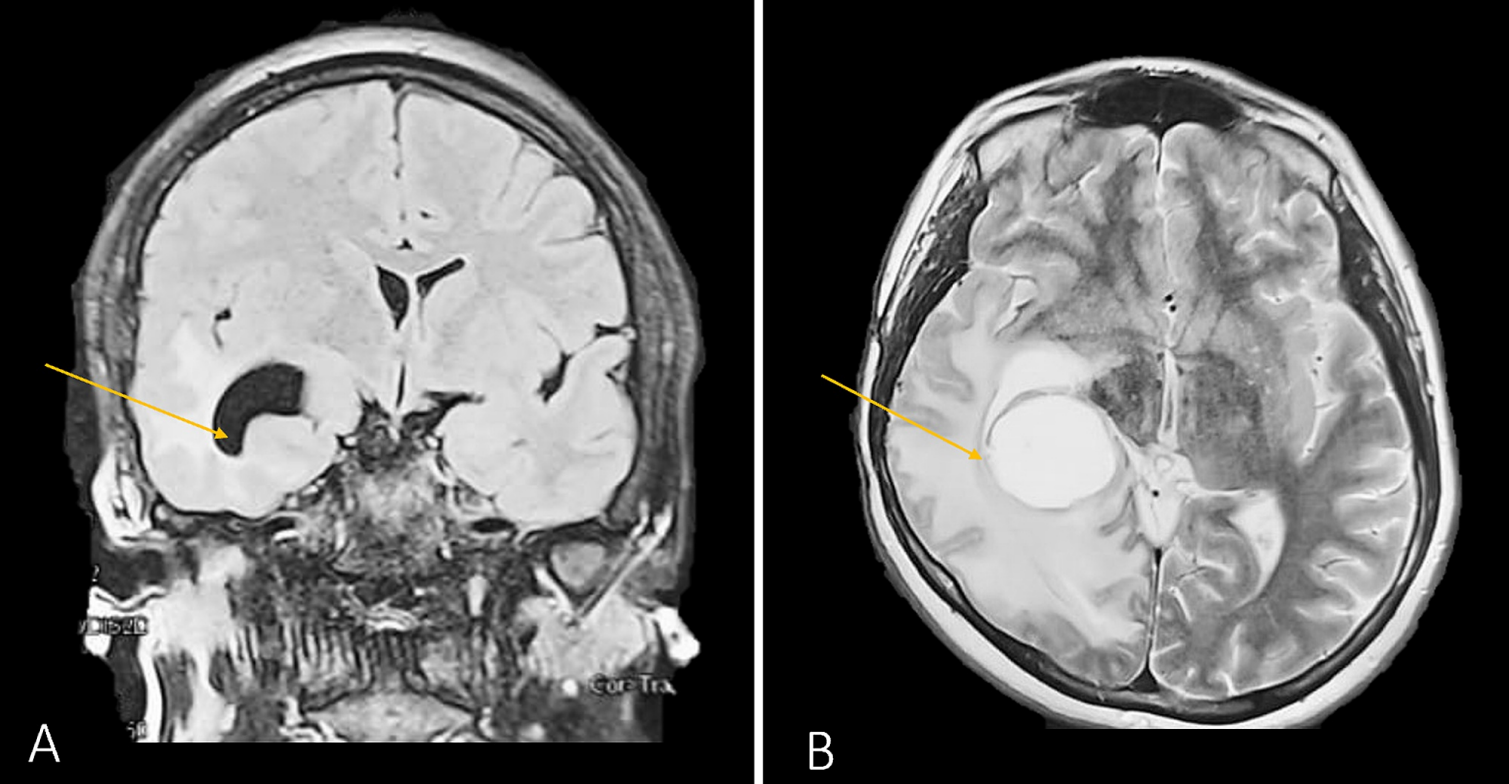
Cranial MRI after the third surgery Coronal (image A) and axial (image B) T2-weighted images, showcasing a right-sided partial lobectomy with sparing of the superior temporal gyrus (yellow arrows) MRI: magnetic resonance imaging
